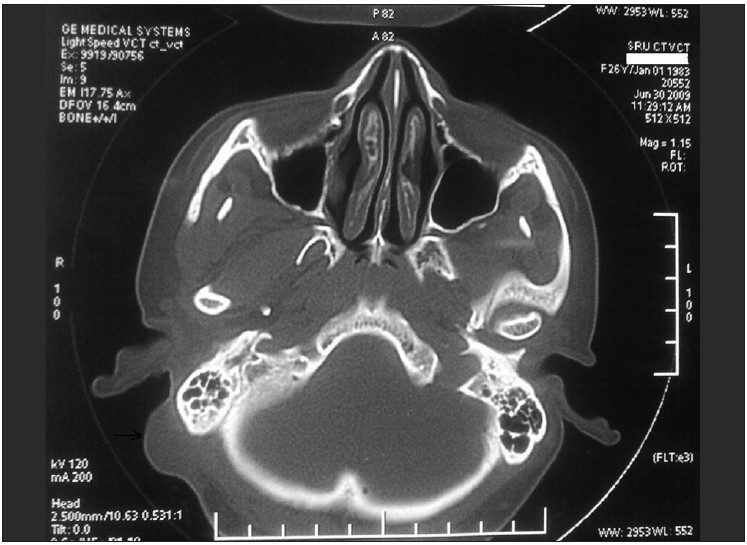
CT scan shows soft tissue mass with uninvolved mastoid bone
